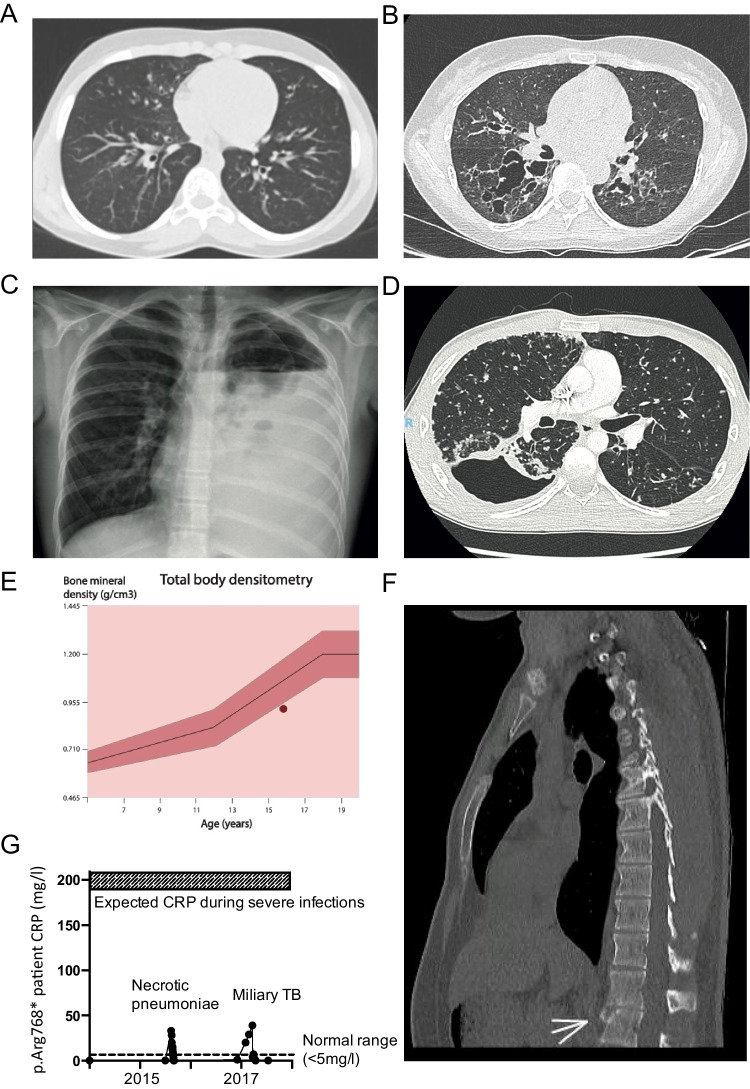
Clinical features of patients P2 (A), P5 (B), and P8 (C–G). A Chest CT scan of patient P2 showing bronchiectasis. B Chest CT scan of patient P5 showing pneumatocele. C Chest X-ray showing parapneumonic effusion and pneumothorax during severe MRSA and *S. pneumoniae* necrotizing pneumonia in patient P8 at the age of 13 years. D Chest CT scan showing bronchopleural fistula during miliary tuberculosis in patient P8 at the age of 15 years. E Total body densitometry showing a bone density below the normal range in patient P8. F Chest CT scan showing signs of an old fracture at L1 in patient P8 at the age of 21 years. G Impaired acute-phase response with a maximum CRP concentration of 33–39 mg/L during episodes of necrotizing pneumonia and miliary tuberculosis in patient P8
